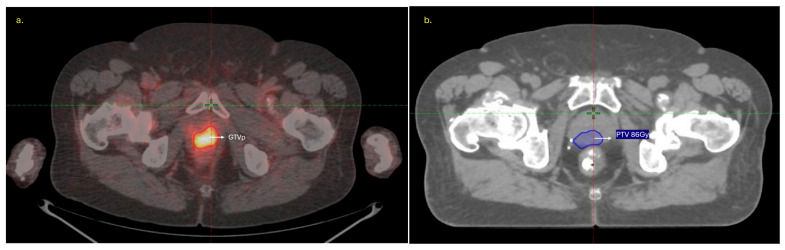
Representative images for the dosiomics analysis showing (a) the GTVp contoured on pre-treatment PSMA-PET/CT and (b) the corresponding intra-prostatic region (planning target volume (PTV) receiving 86 Gy) on the planning CT.
